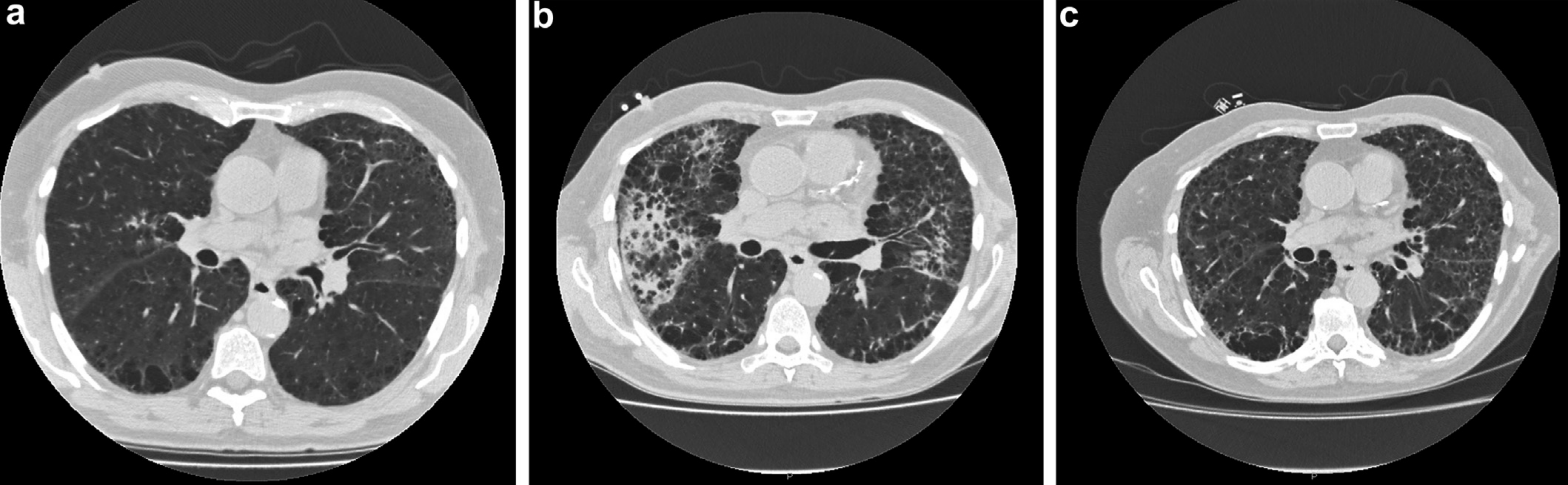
(a) Initial computed tomogram with mild changes, notably extensive emphysema, and bi-basal peripheral–ground-glass changes. (b) Computed tomogram from 4 months later (after initiation of cyclophosphamide), with severe centrilobar and paraseptal emphysema with new inflammatory changes. (c) Improvement in the changes seen in (b), 3 months after cyclophosphamide withdrawal.
